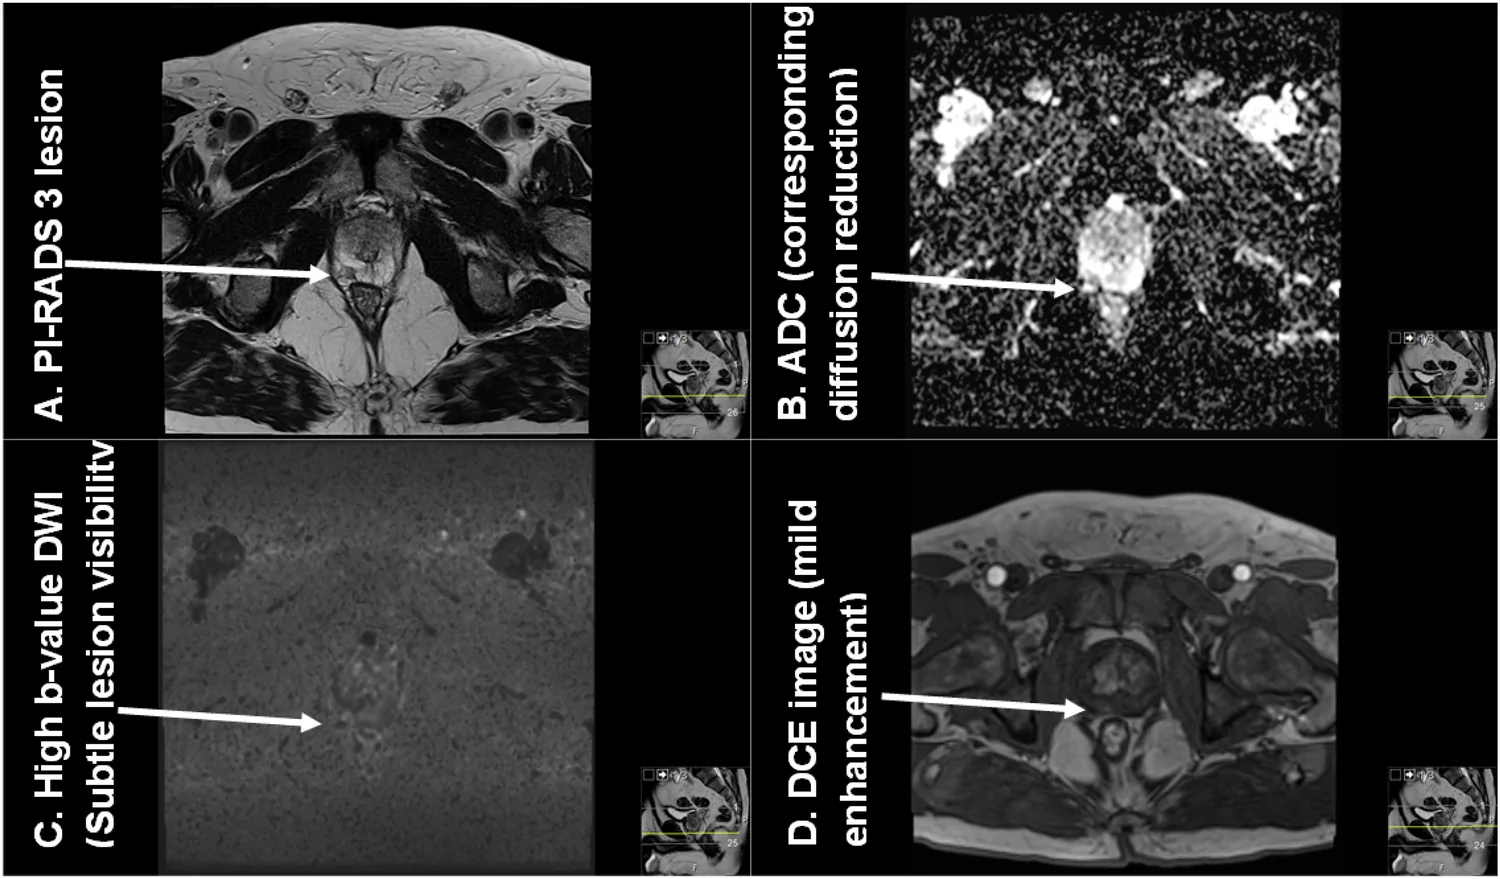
Representative PI-RADS 3 lesion in the peripheral zone. A Axial T2-weighted image demonstrates a focal hypointense lesion (arrow). B ADC map shows a corresponding low signal. C High b-value DWI demonstrates mild hyperintensity. D Dynamic contrast-enhanced imaging demonstrates mild post-contrast enhancement. This represents a typical equivocal lesion where selective GBCA administration may improve diagnostic confidence
